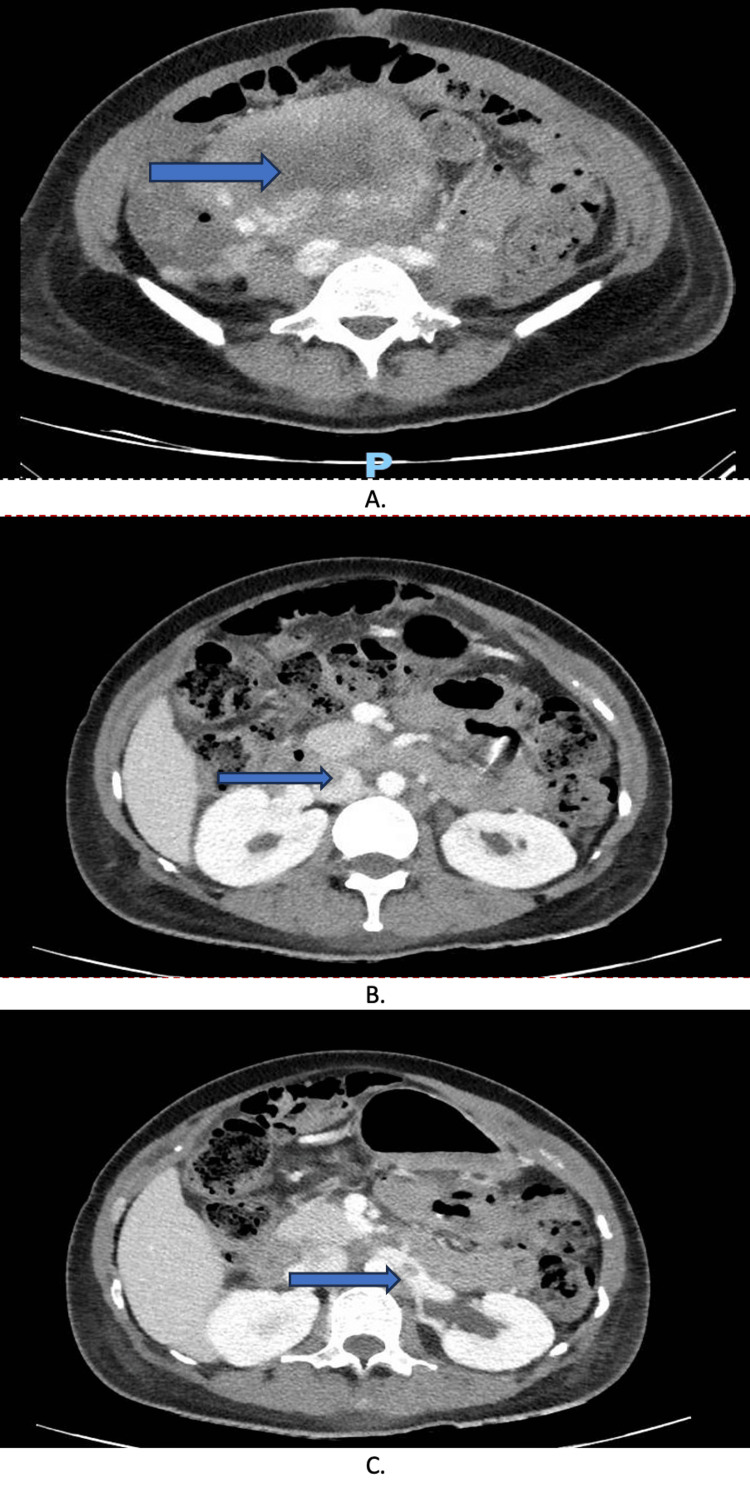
Abdominal CT scan A: Abdominal hematoma, B: Inferior cave vein thrombosis, C: Left renal vein thrombosis
